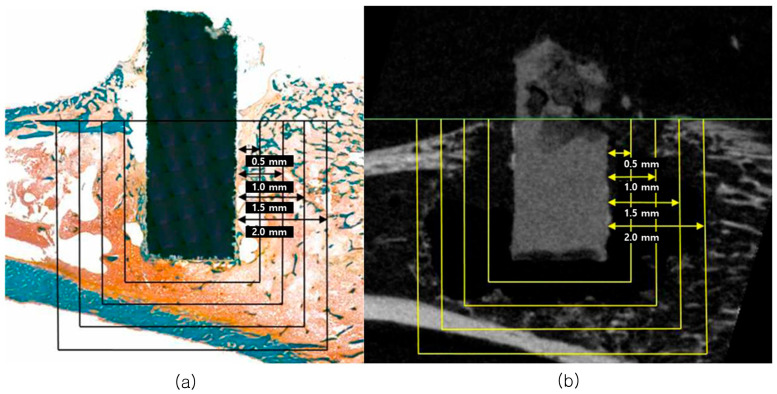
Bone amount of tissue section (a) and radiographic image (b) based on ROI range at 0.5 mm, 1.0 mm, 1.5 mm, and 2.0 mm apart from the innermost point of the cylinder magnesium implant surface.
